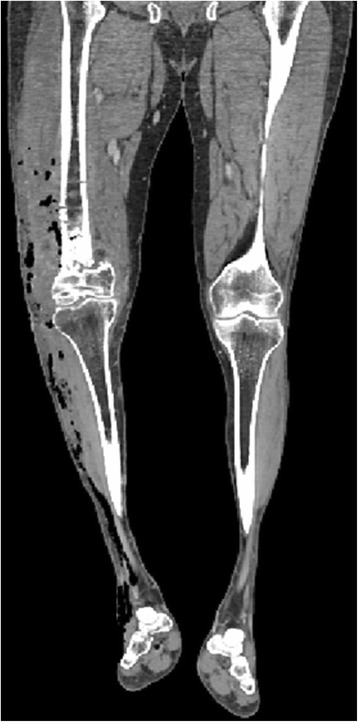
A CT scan showed the subcutaneous emphysema of the leg
